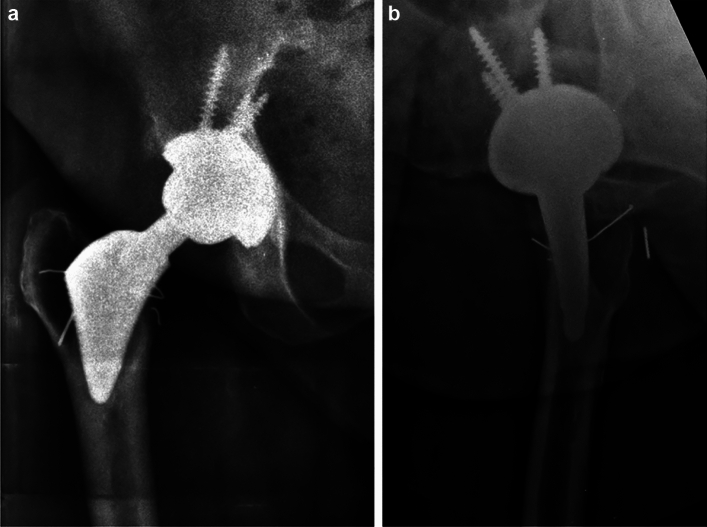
a, b Radiographs of Proxima at 13.5 years, performed for post-traumatic arthritis. a AP view demonstrating circumferential stem osseointegration. Note the preservation of proximal metaphyseal bone density. b Lateral radiograph demonstrating circumferential osseointegration and positive metaphyseal bone remodeling.
